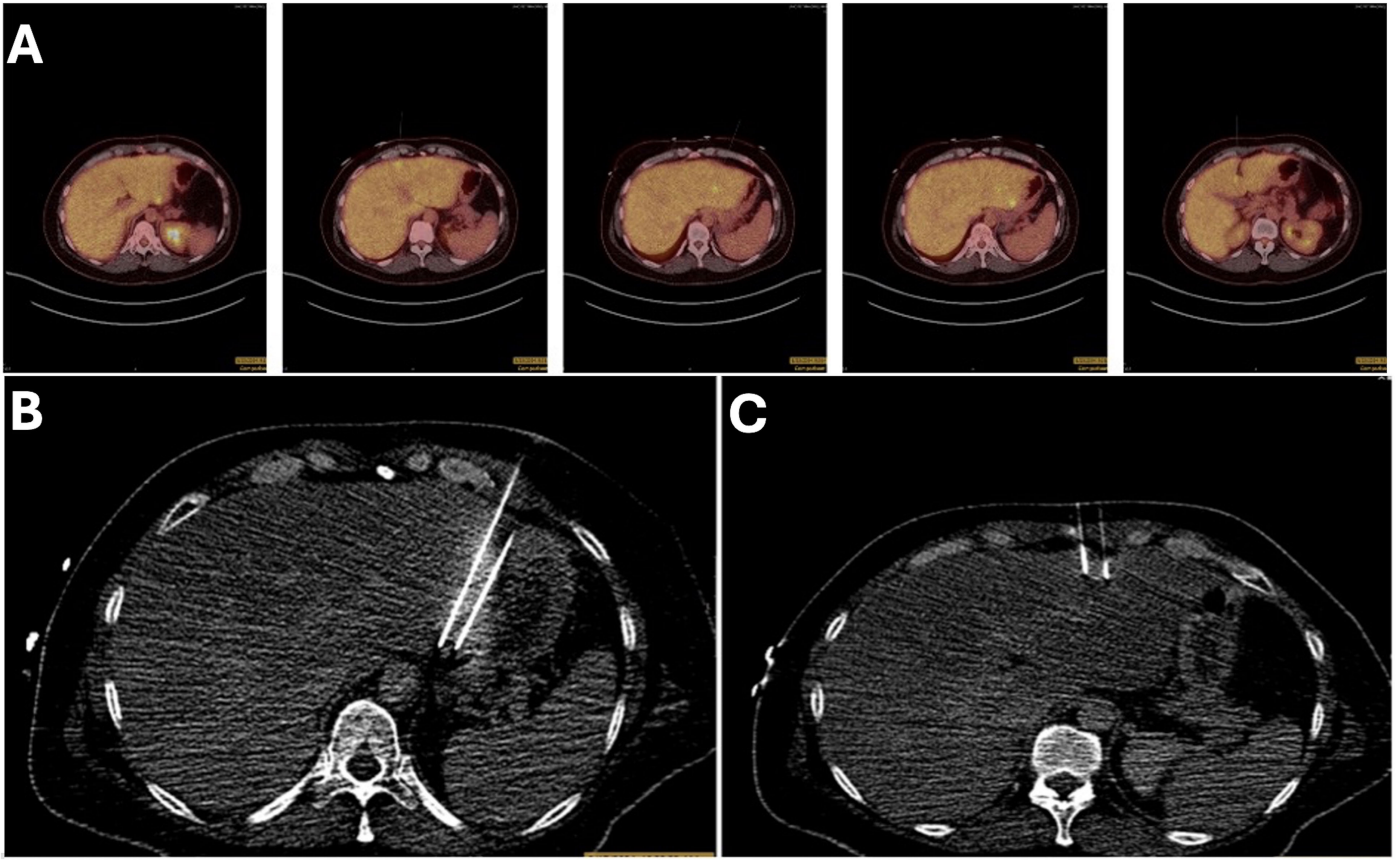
PET/CT scan (A) Pre PEF demonstrating 6 FDG avid lesions in the liver. (B) post PEF demonstrating resolution of 5 lesions within the left hepatic lobe.
